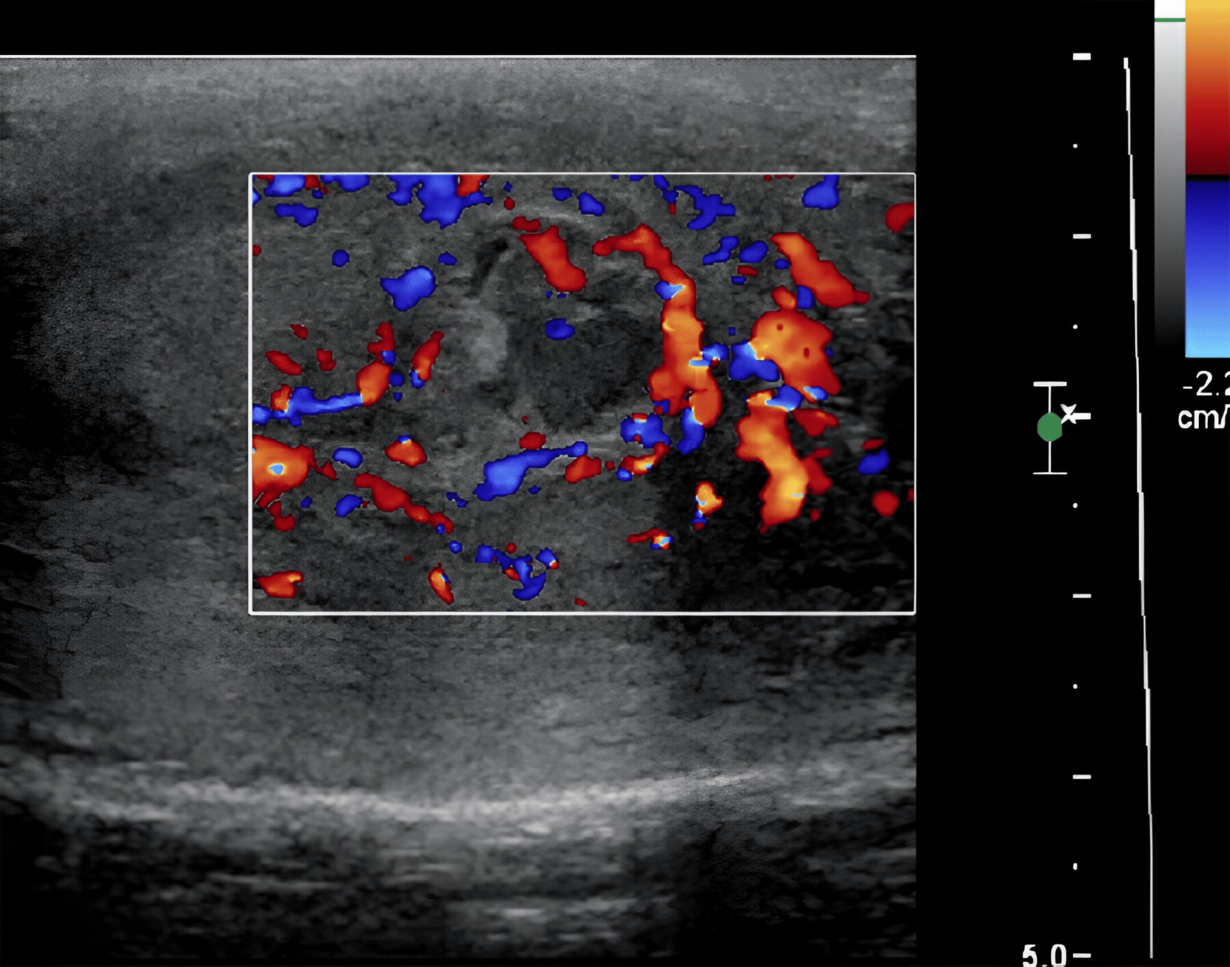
Ultrasonographic Doppler imaging of left epididymis showing increased vascularity and heterogeneous echogenicity in the left epididymal tail (white square) consistent with epididymo-orchitis.
